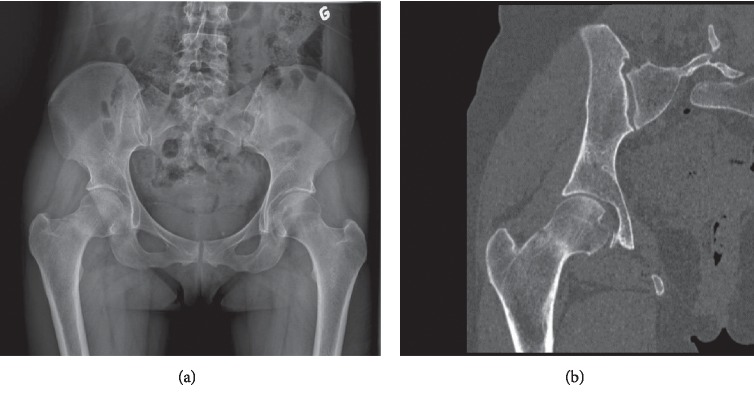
(a) AP pelvic X-ray showing a stress fracture of the right femoral neck. (b) Frontal view on CT scan of the right hip confirming the femoral neck fracture.
